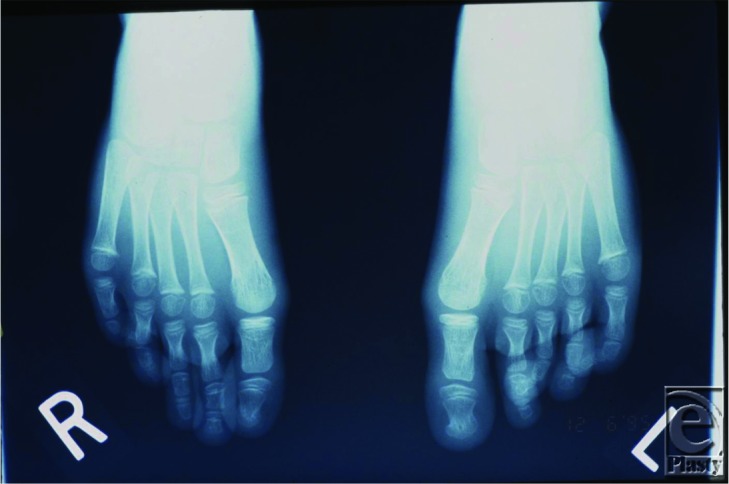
Radiograph of the feet showing osteohypertrophy of the first metatarsal.
